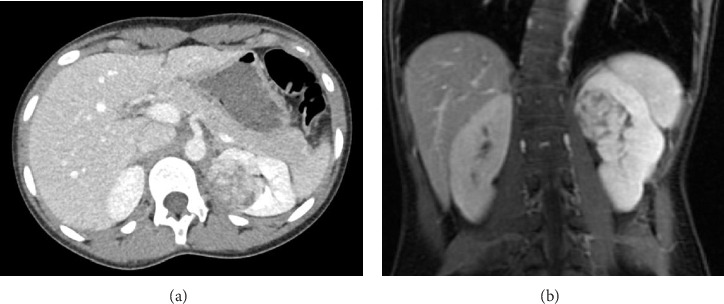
A mass in the upper pole of the left kidney shown by CT imaging (a). MRI imaging (b) showed a heterogeneous mass within the upper pole of the left kidney measuring 3.8 × 4.1 × 3.6 cm. The lesion demonstrated intermediate to high signal on T2-weighted imaging, intermediate to low signal on T1-weighted imaging, and enhancement similar to the kidney with multiple irregular areas of decreased signal present throughout the lesion. No other focal renal lesions, no hydronephrosis, and no ureteral dilation were noted, and the inferior vena cava was patent.
